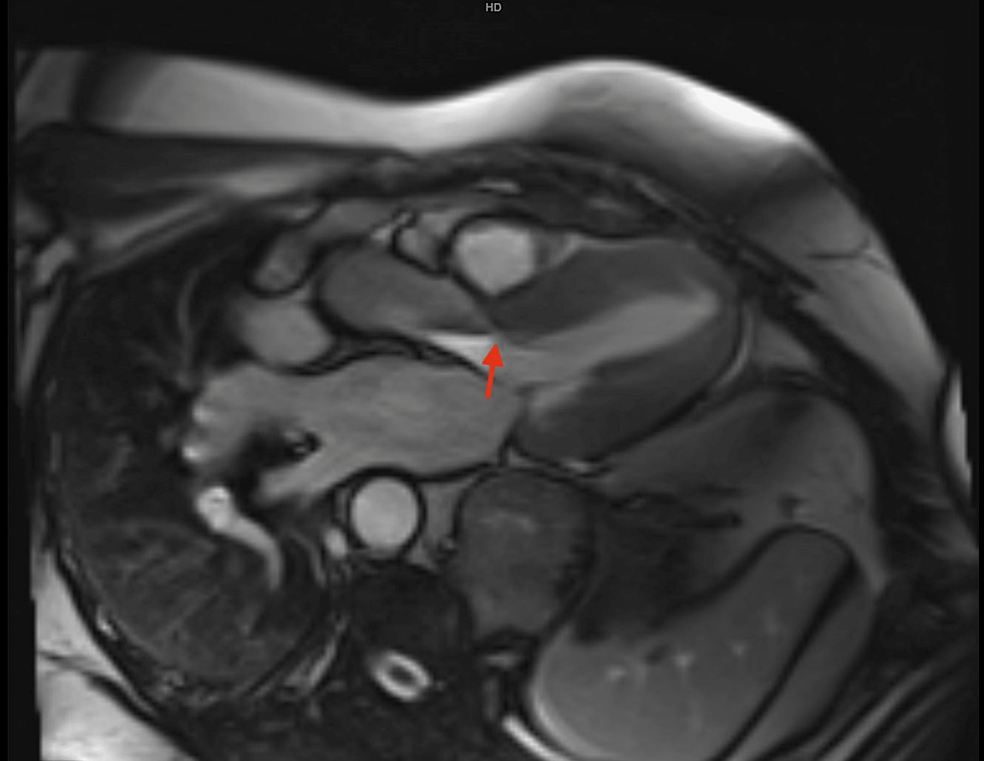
Cardiac MRI axial slice showing subaortic membrane (red arrow).
